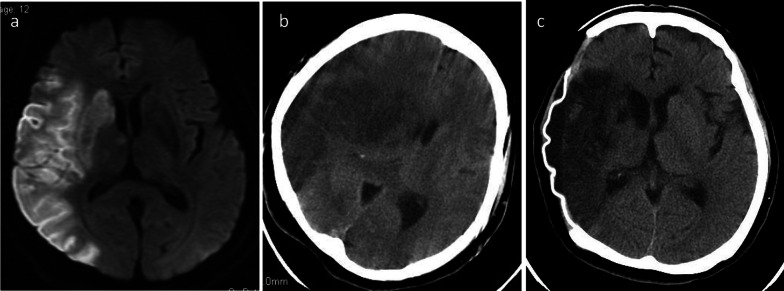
Brain MRI shows massive cerebral infarction of the right middle cerebral artery area (a). Brain CT shows diffuse brain edema resulting in brain herniation (b), and no cerebral edema after external decompression (c)
